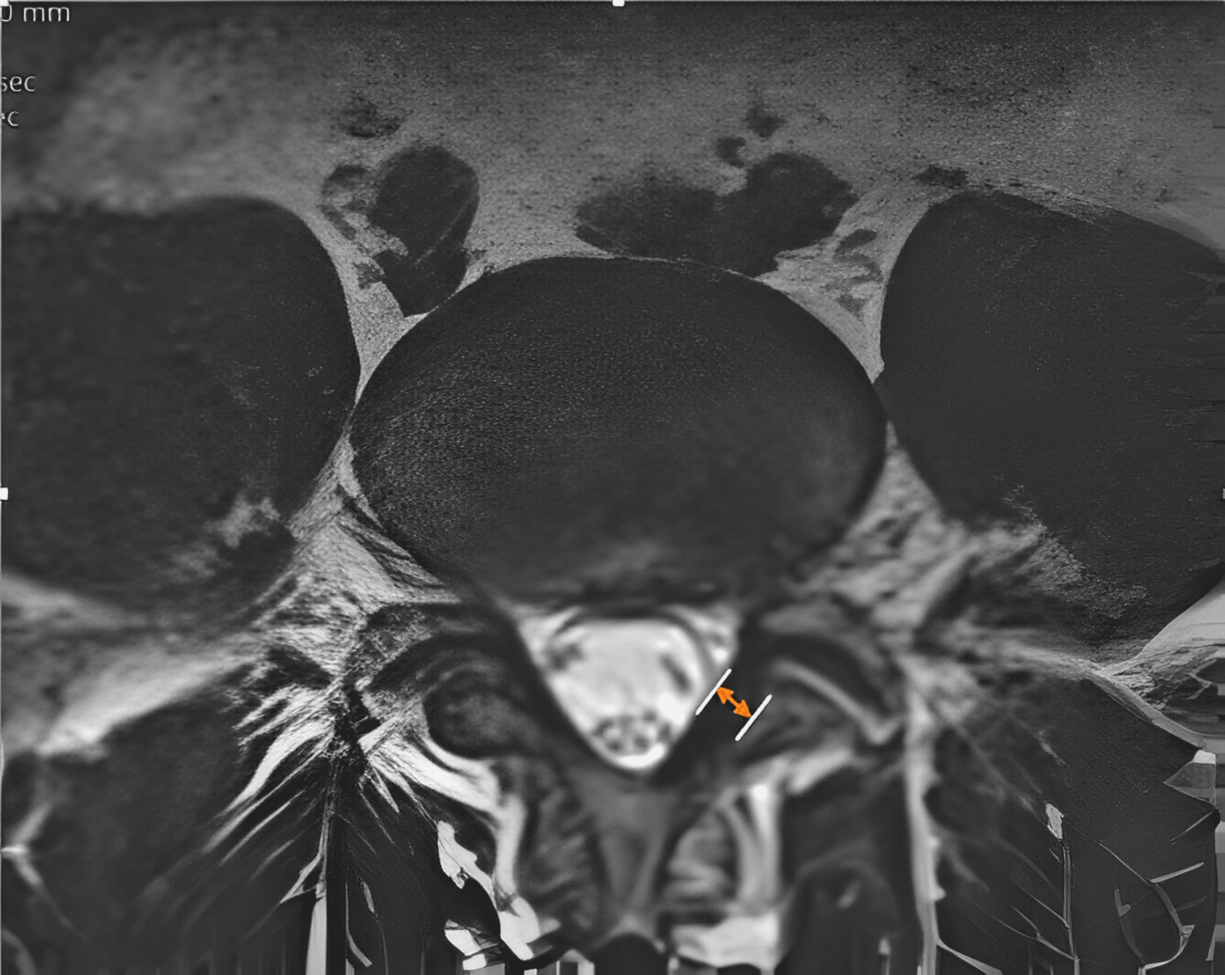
Measurement of the ligamentum flavum thickness on axial T2-weighted MRI image at the level of the facet joint MRI: magnetic resonance imaging Source: original image
